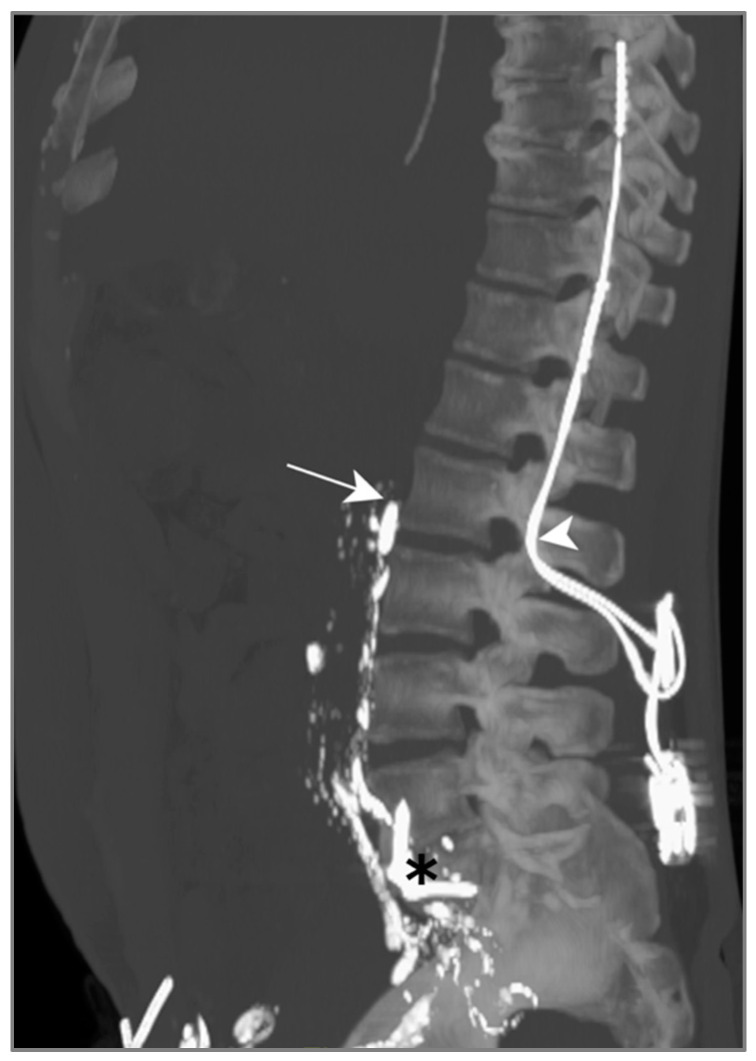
Patient B. The maximal-intensity projection of the CT scan reconstructed in the sagittal plane. The progression of Lipiodol in the prevertebral lymphatic chains is demonstrated up to the level of the 2nd lumbar vertebra (white arrow). The location of a spinal neurostimulator (white arrowhead) and anterior lumbar arthrodesis material (black asterisk), both anteriorly placed, are highlighted.
